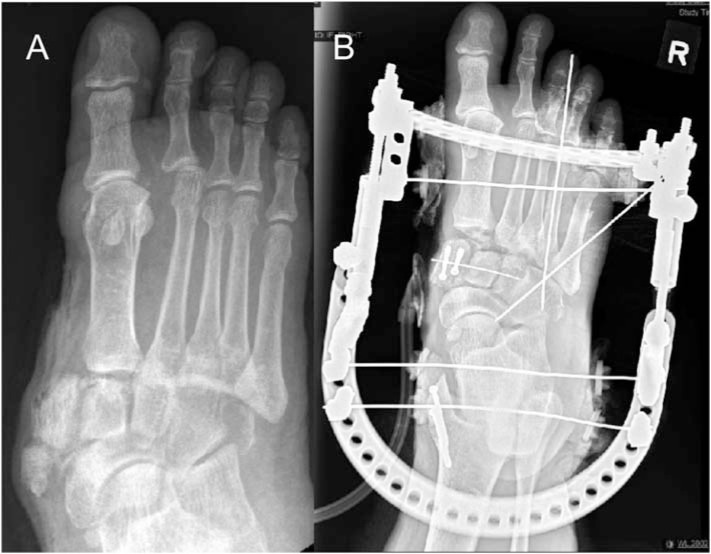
A: Radiograph showing right foot Lisfranc dislocation with cuneiform and cuboid fractures.B: Radiograph post-external fixation and midfoot arthrodesis.
